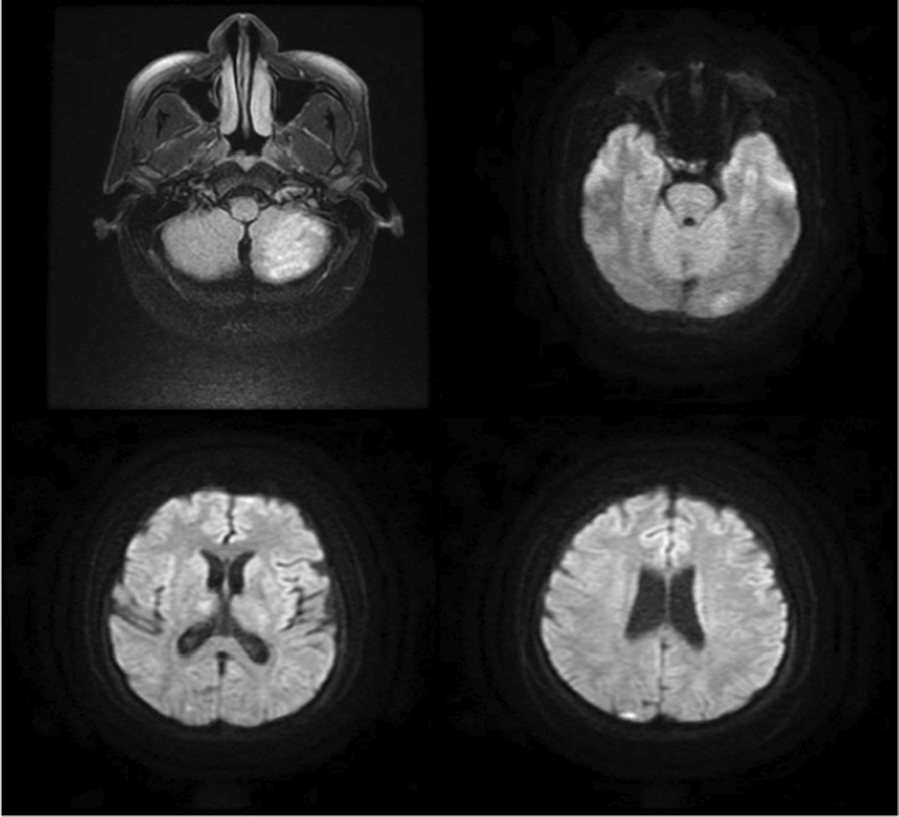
MRI brain axial DWIs show left inferior cerebellar, left occipital, and right thalamic subacute infarcts and right occipital acute cortical infarct
